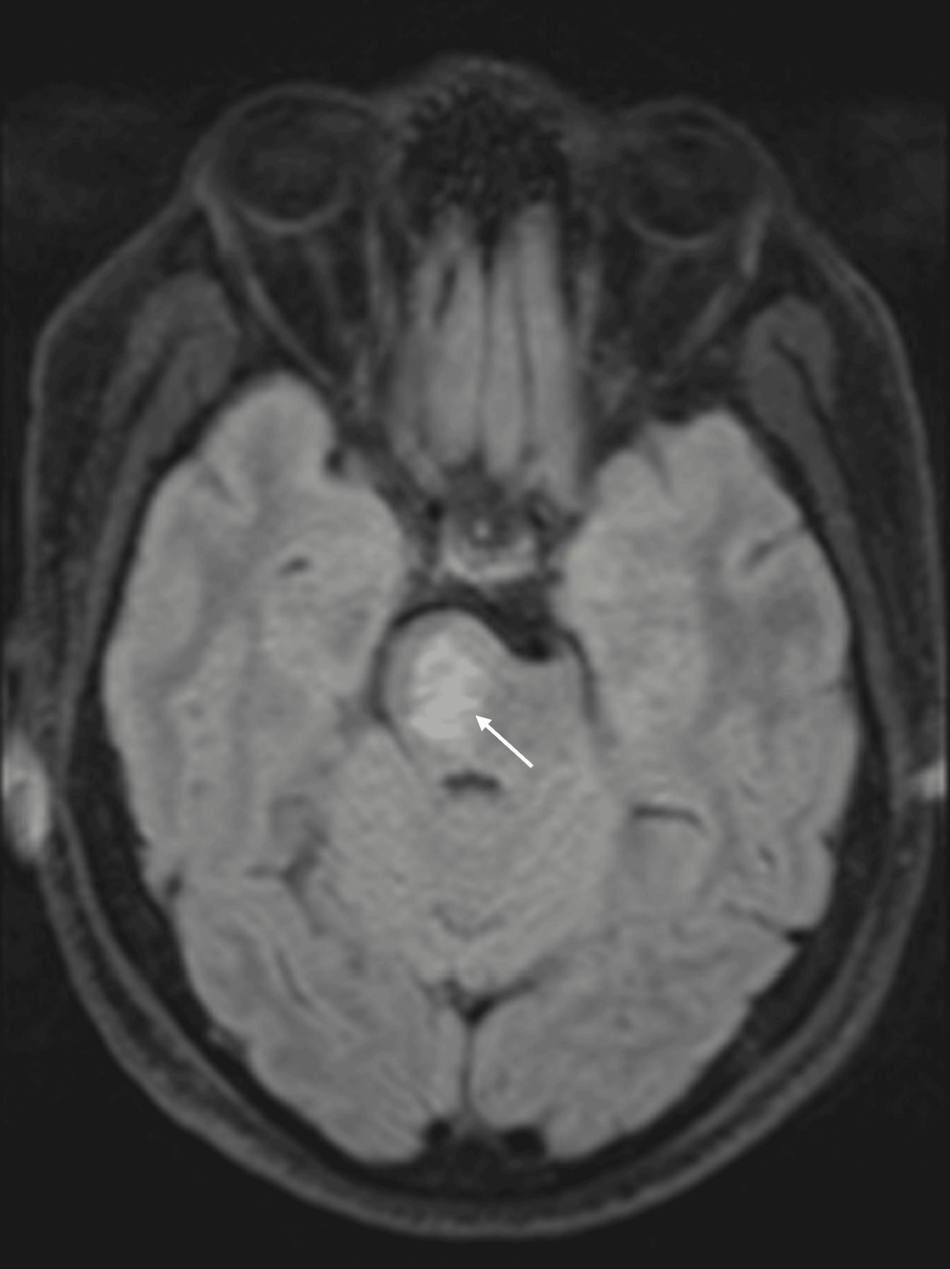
Brain MRI demonstrating an acute ischemic lesion in the right pons. Axial T2-FLAIR (fluid-attenuated inversion recovery) sequence showed a hyperintense lesion in the right aspect of the pons (arrow), compatible with an acute ischemic event and correlating with the patient’s left-sided motor deficits.
